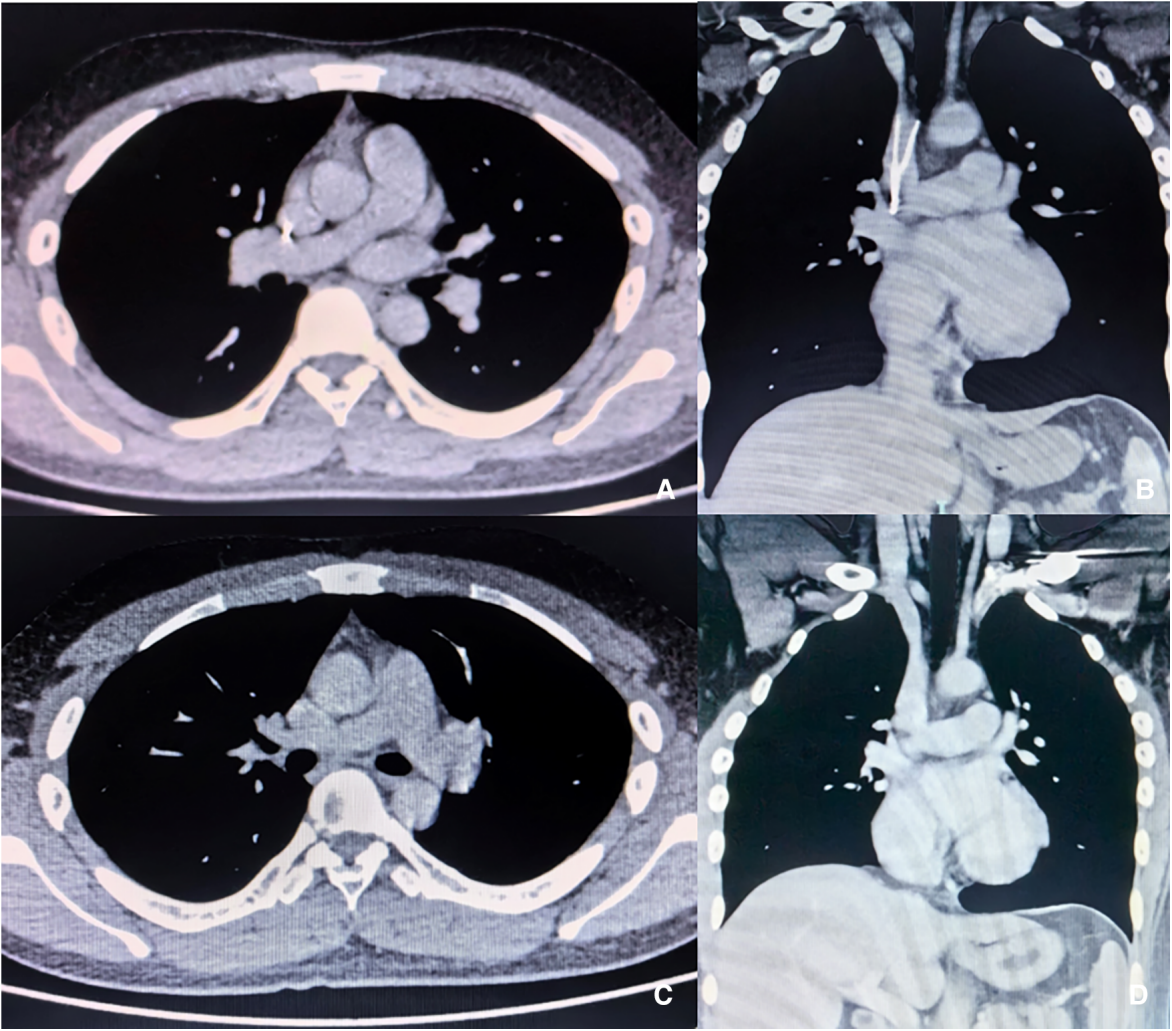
Enhanced CT images before and after procedure. (A,B) The filter retraction hook entered SVC wall, and the tip was close to the right pulmonary artery. (C,D) No haematoma, dissection or cardiac fluid accumulation in the superior vena cava or right pulmonary artery after filter removal.
